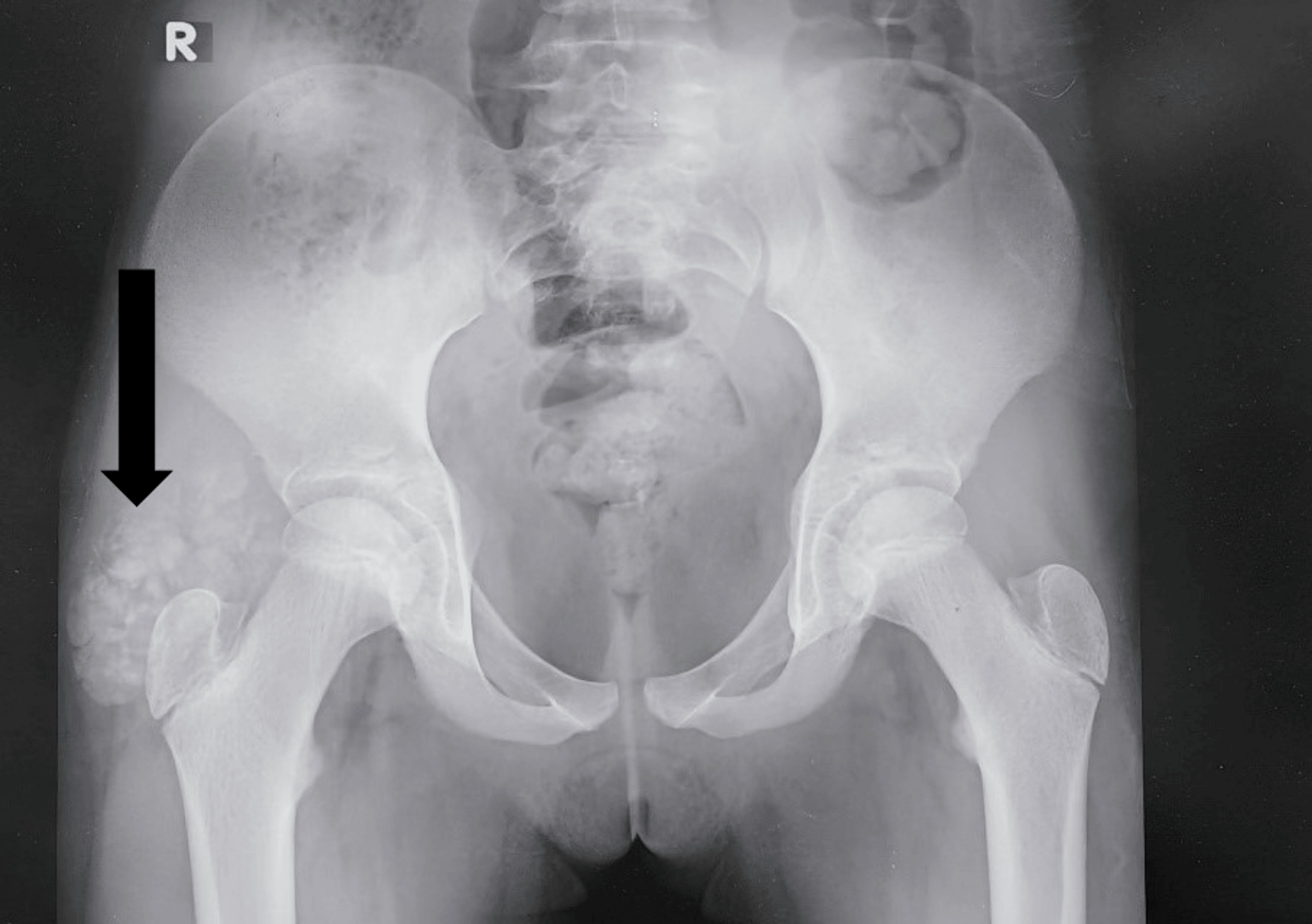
Anteroposterior (AP) X-ray of both hips shows right hip periarticular calcification (arrow).
